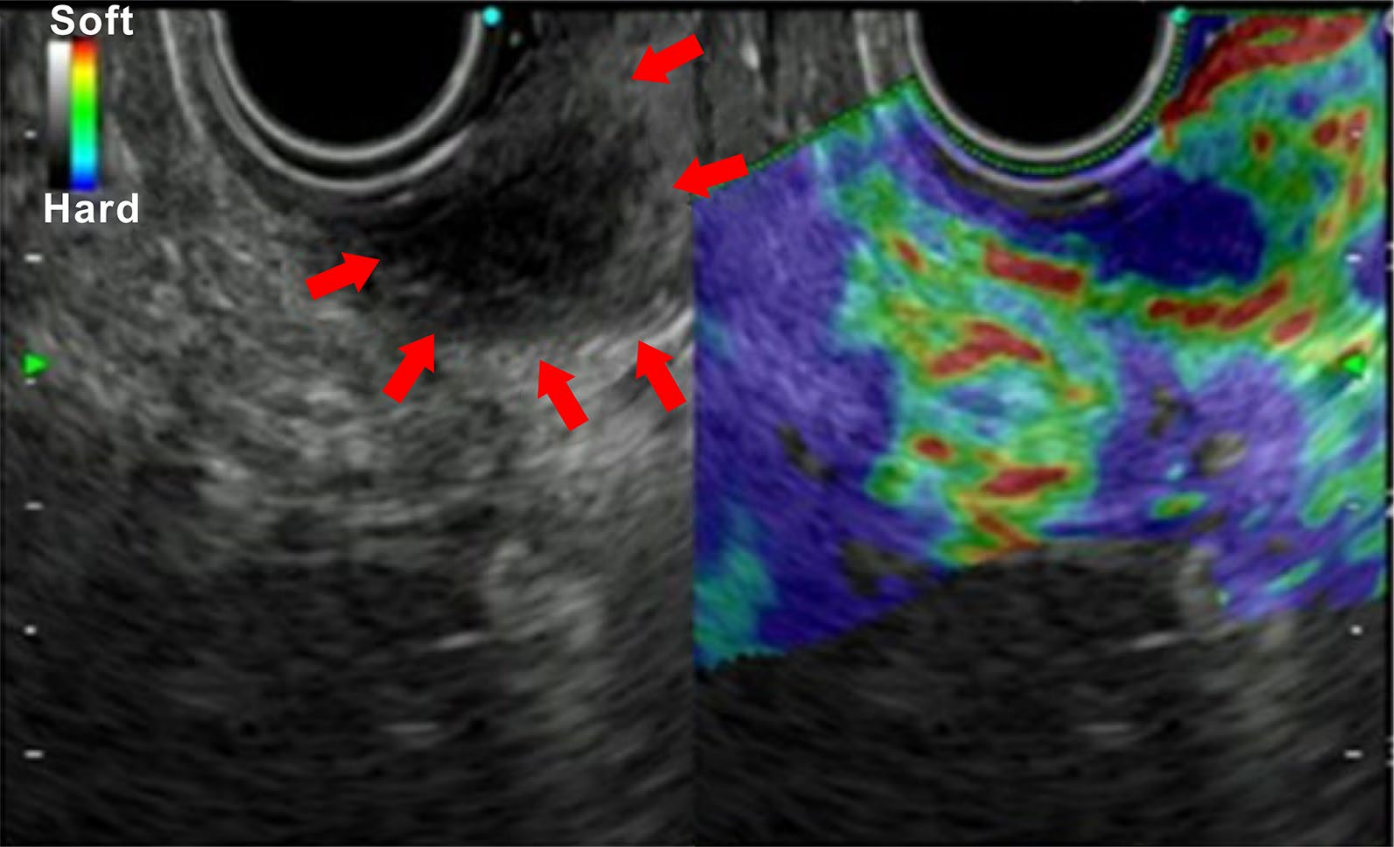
Ultrasound-guided endoscopic examination. An ischemic tumor of submucosal origin was suspected
